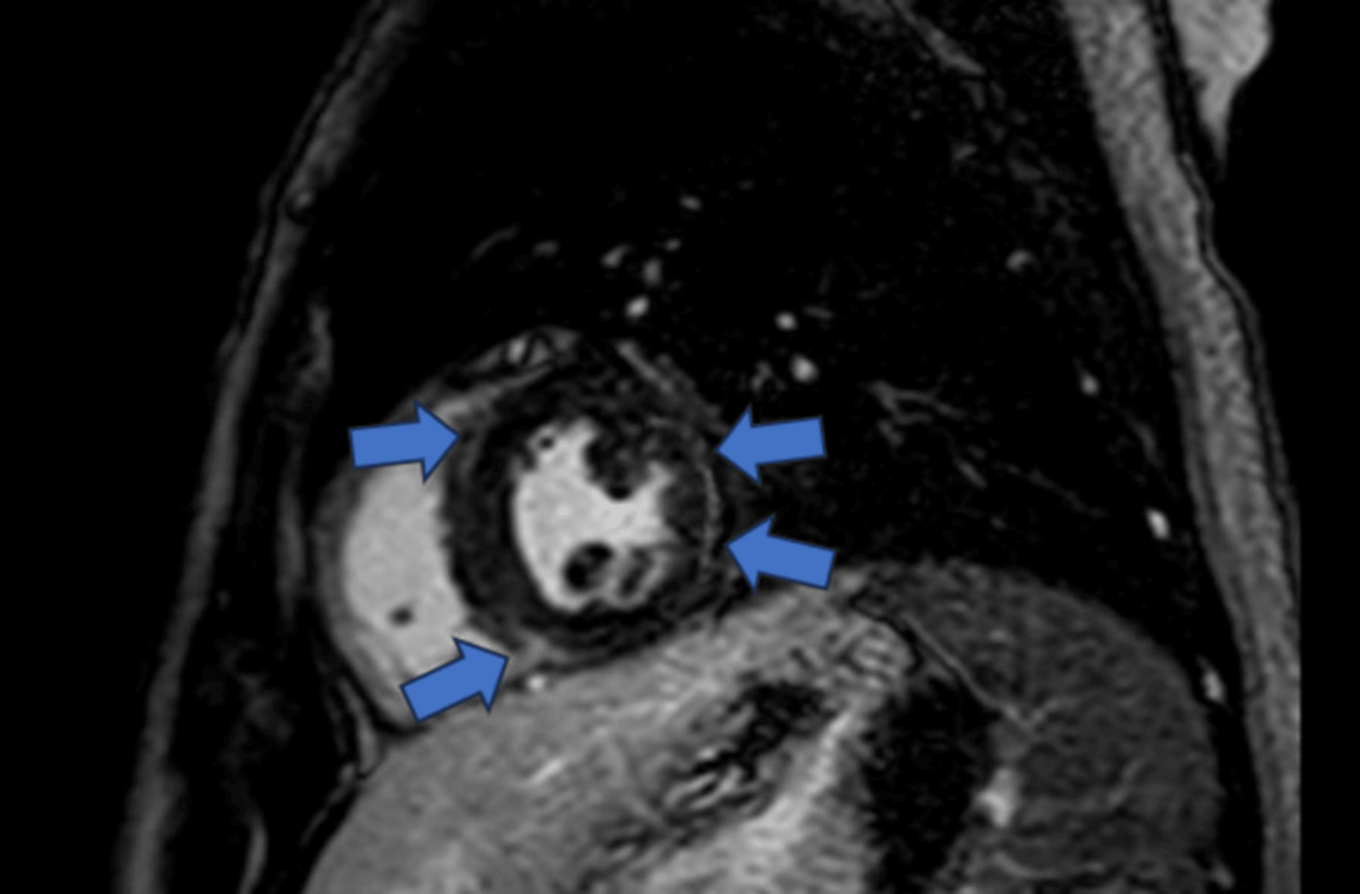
Cardiac Magnetic Resonance Imaging (CMR) Short-axis view demonstrating diffuse subepicardial late gadolinium enhancement (LGE) involving the lateral wall of the left ventricle (blue arrows), consistent with active inflammatory myocarditis. The imaging findings fulfill the Lake Louise criteria for the diagnosis of acute myocarditis [8,9].
